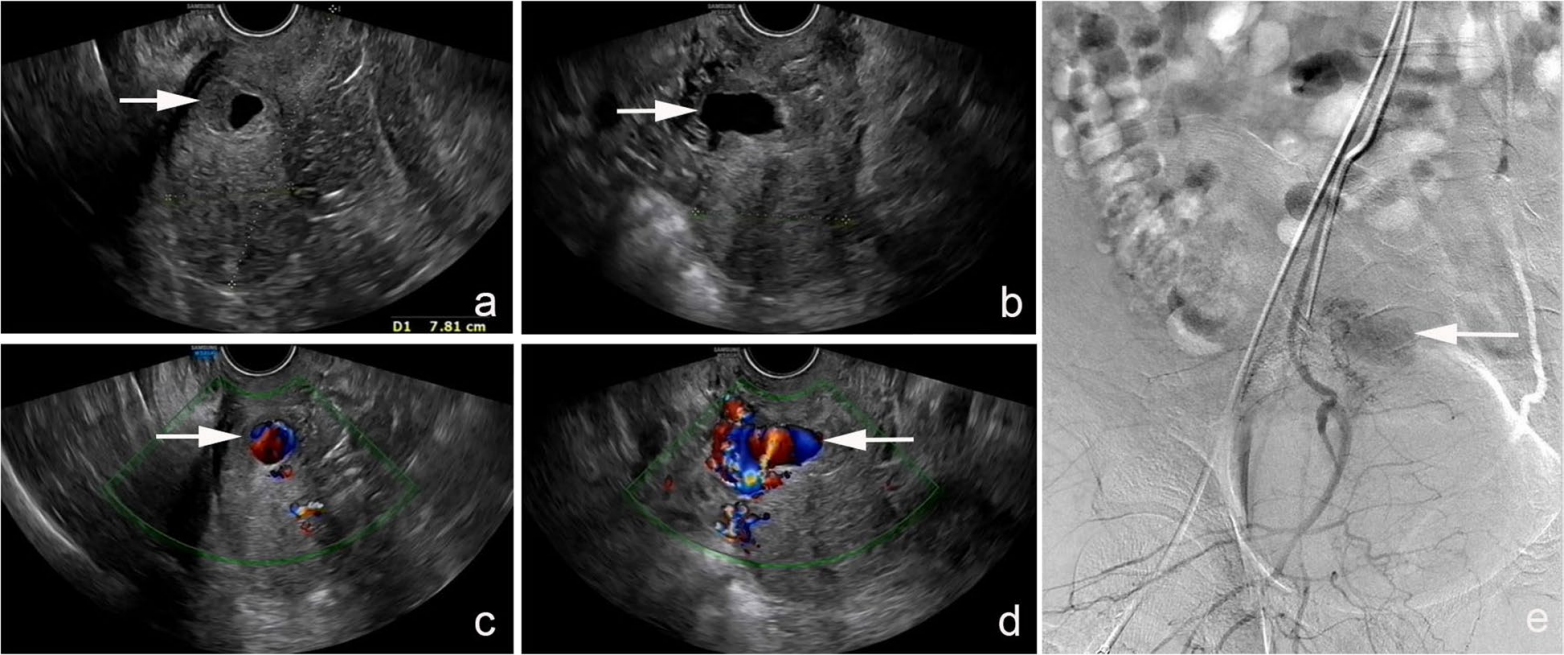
Doppler ultrasound and DSA images of uterine artery pseudoaneurysm. (a, b, c, d) TVUS: a 2.7 cm × 1.2 cm × 1.7 cm cystic mass is seen on the right side of the isthmus of cervix, with a clear boundary and irregular shape; the mass shows good perfusion. The low echo area with uneven thickness is seen around the liquid area, which is about 0.8 cm in the thicker area. (e) DSA: abnormal concentration of contrast medium is found
